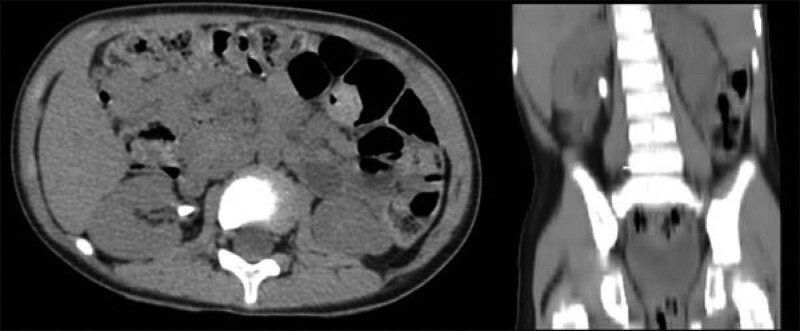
Sagittal and coronal view of lower abdominal CT before operation (Case 1). CT = computed tomography.
